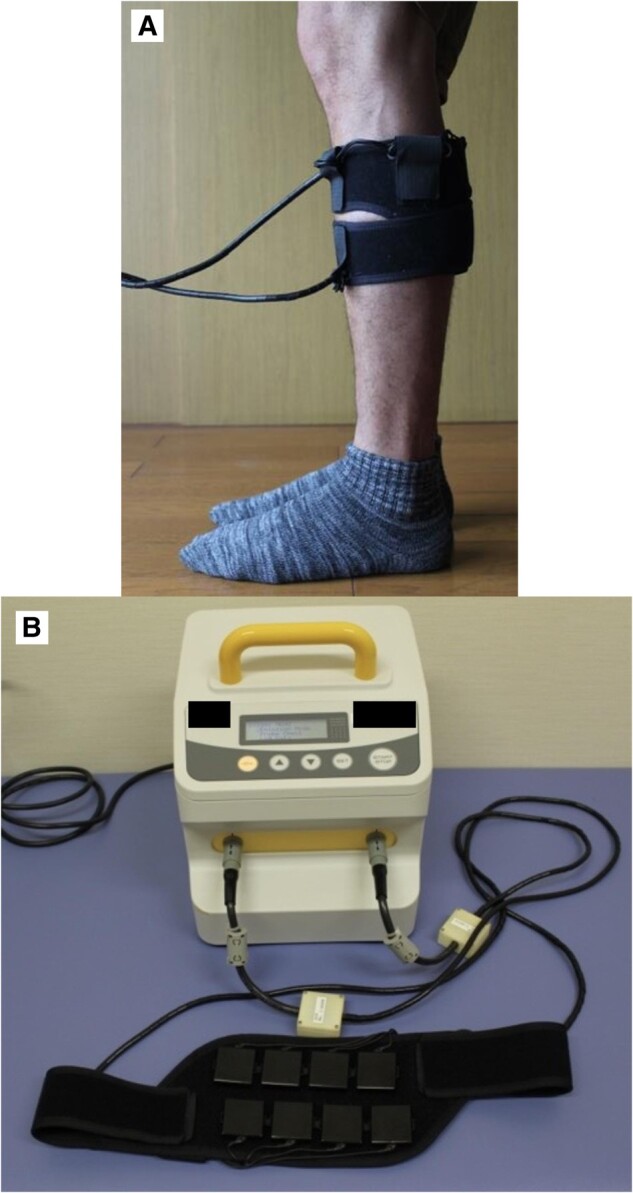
Low-intensity pulsed ultrasound. The application of the low-intensity pulsed ultrasound device on a model’s leg (*A*) and the low-intensity pulsed ultrasound device set (*B*).
